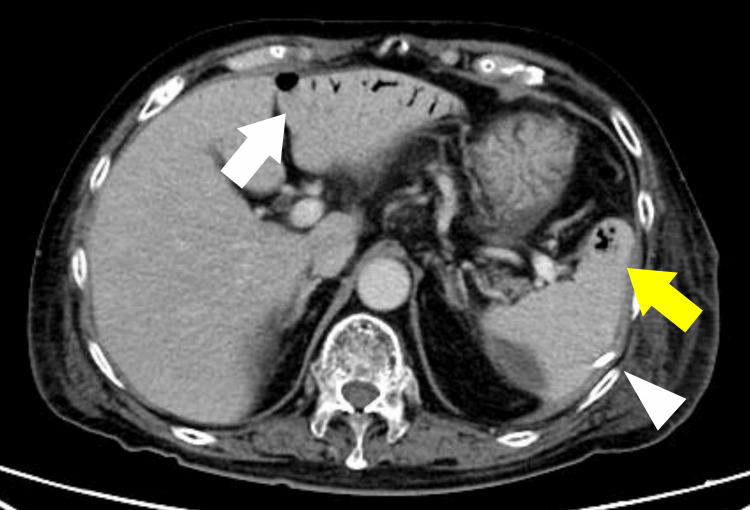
CT findings after percutaneous drainage on hospital day 3. After placement of a pigtail catheter, the perisplenic abscess had decreased in size (white arrowhead). In contrast, a new gas-containing abscess had appeared within the splenic parenchyma, consistent with an intrasplenic abscess (yellow arrow). Portal venous gas was also seen in the liver (white arrow).
